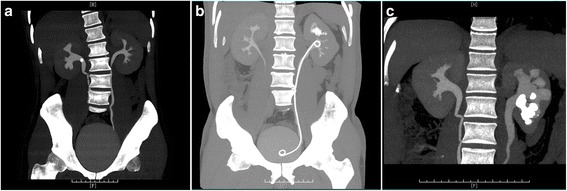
The demonstration of the simplest calculus to the most complicated renal stone presented by computed tomography of urography. a The R.I.R.S. score was calculated as 4, the simplest calculus. b The R.I.R.S. score was calculated as 7. c The R.I.R.S. score was calculated as 10, the most complex situation
